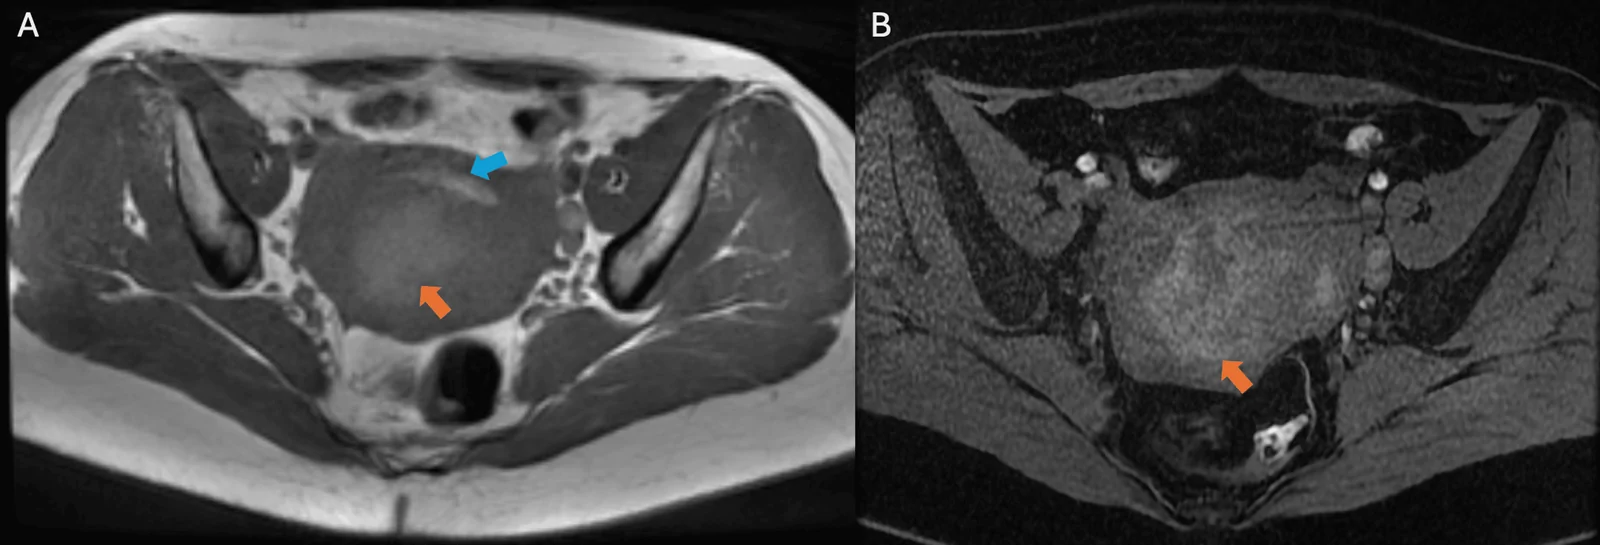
MRI features of the uterine tumour. Axial T1-weighted and T1 fat-saturated images (A, B) show a small hematometra (blue arrow, A) and intralesional haemorrhage (orange arrows).
